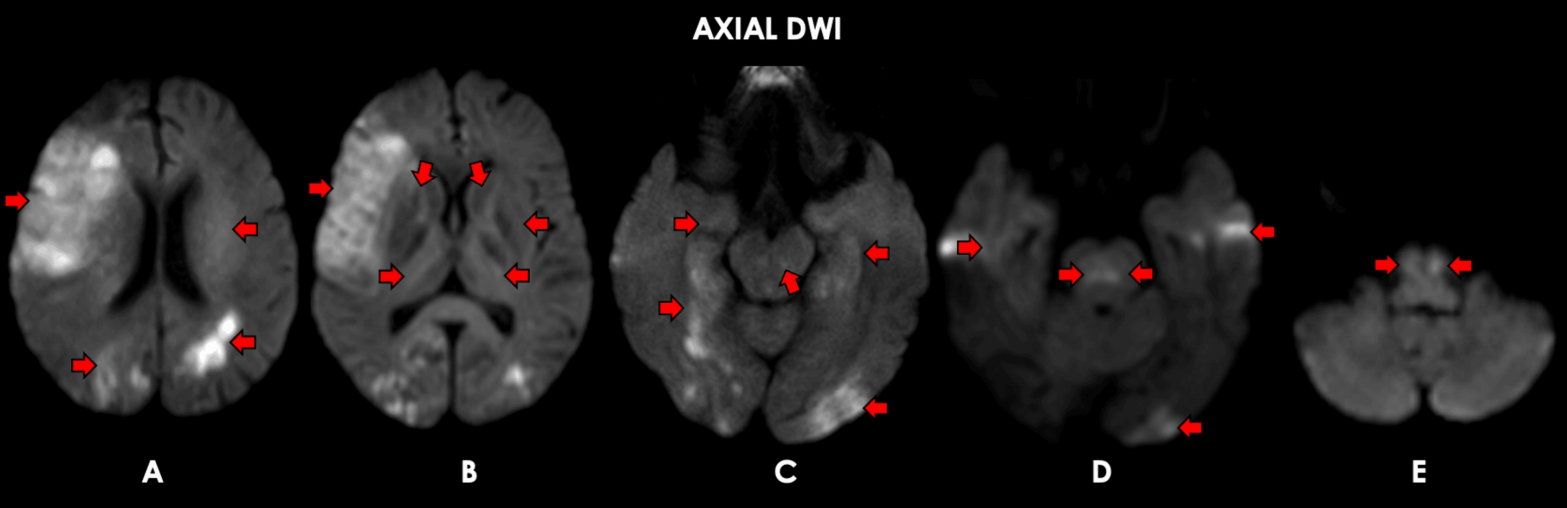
Representative cranial MRI sequences for patient #2. Representative axial brain MRI (DWI sequence) images show restricted diffusion at the sites of the previously described FLAIR-hyperintense lesions in Figure 3, including the bilateral frontoparietal cortical, subcortical, and periventricular regions (A); bilateral insular cortex, caudate nuclei, thalami, and lentiform nuclei (B, C); bilateral medial temporal lobes, midbrain, and pons (D); and bilateral medulla (E). FLAIR: Fluid-attenuated inversion recovery; DWI: Diffusion-weighted imaging.
